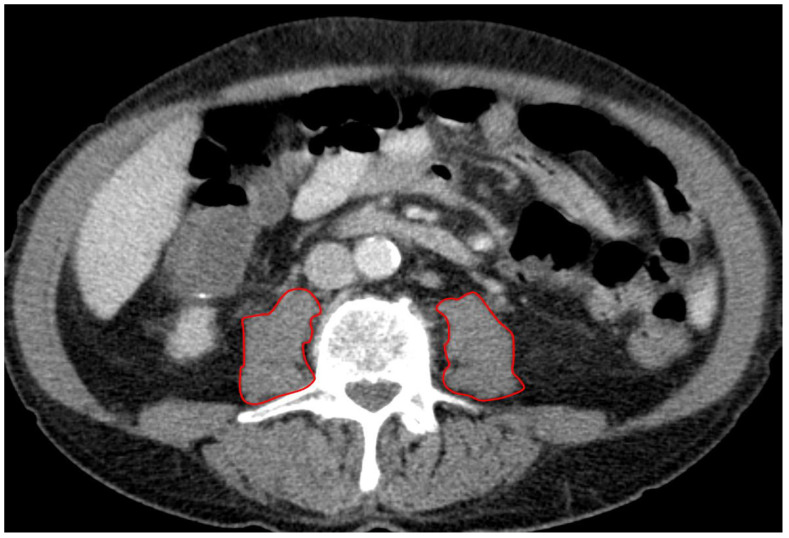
Radiological measurement of PMA. Figure 1 demonstrates the measurement of left and right PMA by manually tracing the perimeter of the psoas muscles on an axial CT scan. The combined areas of the left and right muscles, adjusted for BSA, provided the PMAi.
